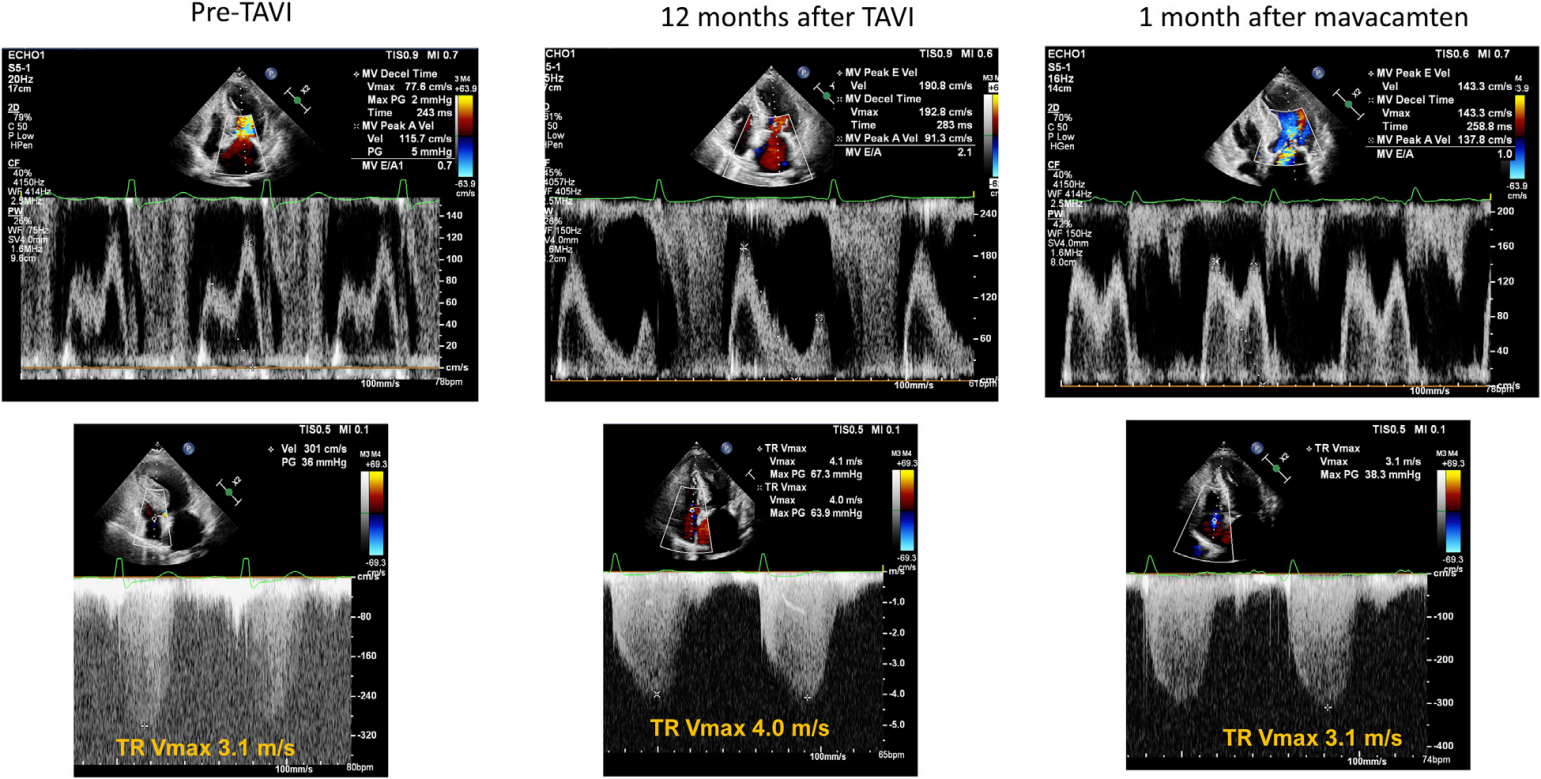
Serial Changes in Doppler Tracing of Mitral Inflow and Tricuspid Regurgitation Jet Before TAVI, an abnormal relaxation pattern of the mitral inflow with mild resting pulmonary hypertension was observed. During follow-up, a restrictive pattern of mitral inflow velocity with significantly elevated pulmonary hypertension was noted, which was reversed with mavacamten treatment. TR = tricuspid regurgitation.
